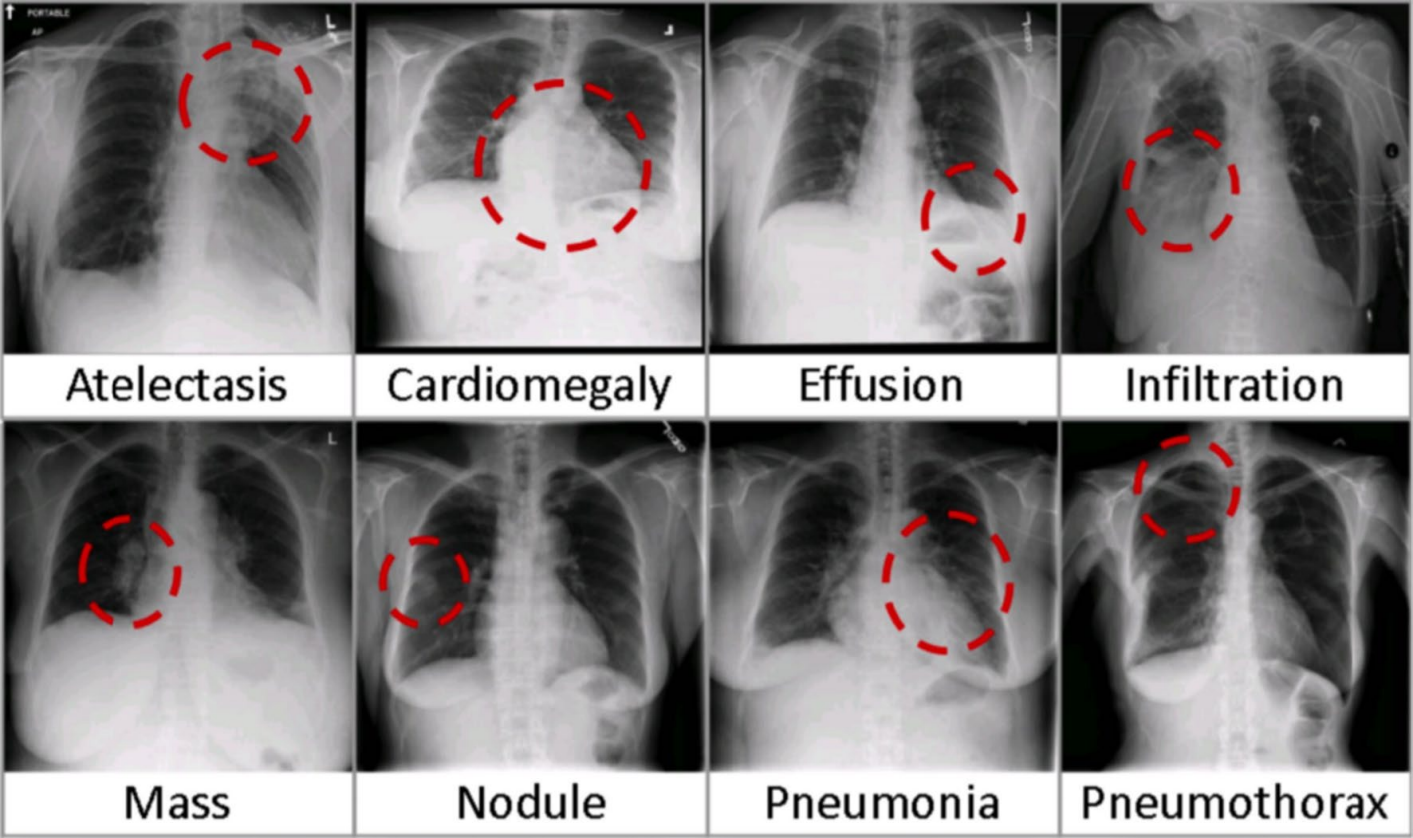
Common thoracic diseases observed in Chest X-ray14 [30]
